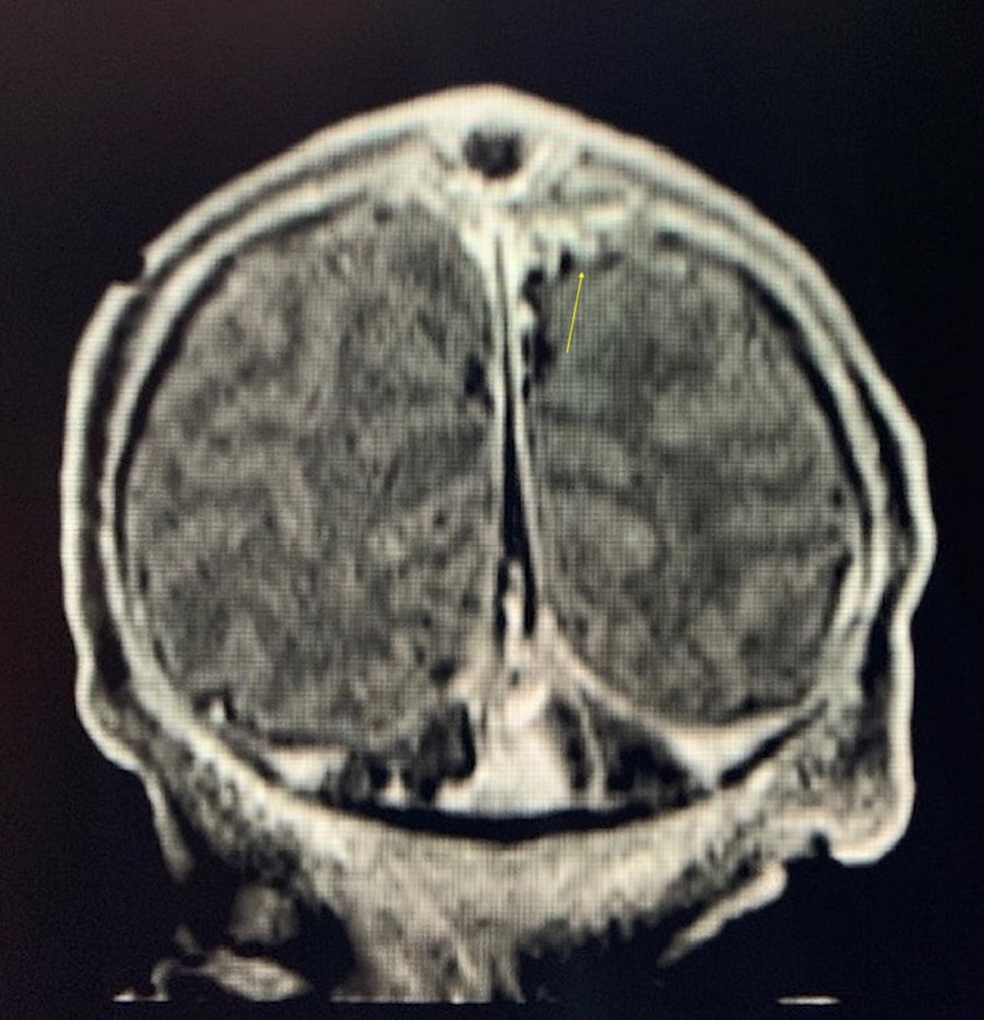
Coronal FLAIR post-contrast MRI. The image is showing fenestrations at the superior sagittal sinus on the left (yellow arrow) at the level of the cystic structure, which is a characteristic finding of APC. FLAIR: fluid-attenuated inversion recovery; APC: atretic parietal cephalocele
